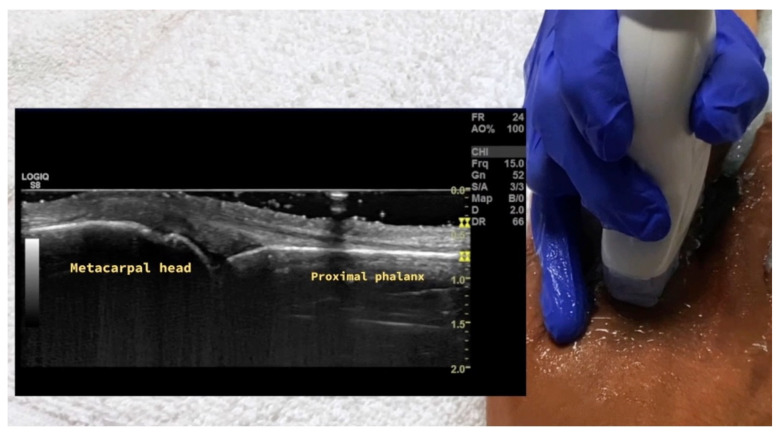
Probe placement and ultrasound of dorsal longitudinal view of MCP joint. MCP = metacarpal phalangeal.
